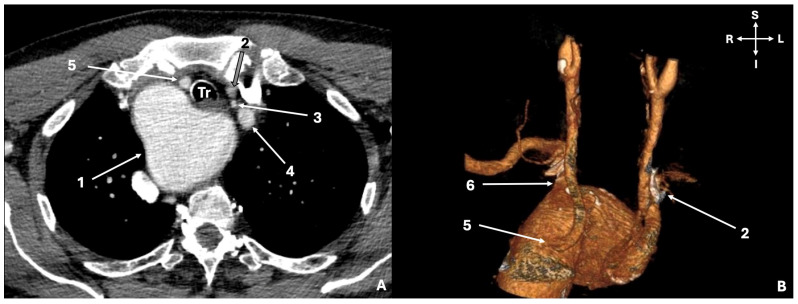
The right-sided aortic arch (RAA). (A). Typical computed tomography scan, (B). Three-dimension reconstruction. 1—RAA with Kommerell’s diverticulum compressing the esophagus and the trachea (Tr), 2—Left common carotid artery (LCCA), 3—Left vertebral artery (LVA), 4—Left subclavian artery (LSA) with aberrant origin from the diverticulum, 5—Right common carotid artery (RCCA), 6—Right subclavian artery (RSA). S—superior, I—inferior, L—left, and R—right.
